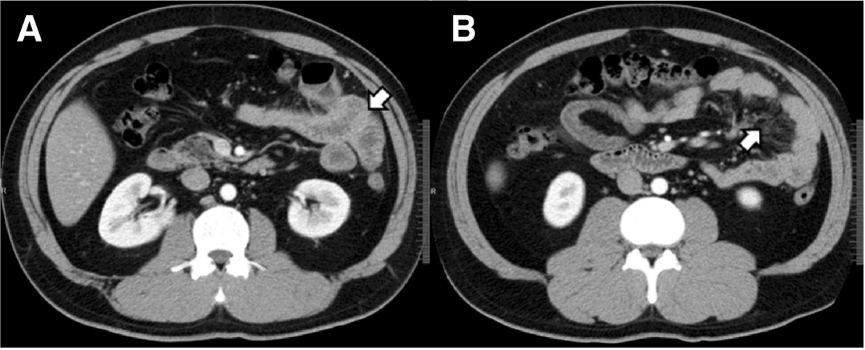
Findings of abdominal computed tomography (CT). A. showing a near-circumferential wall thickening in the jejunum (arrow). B. showing increased density of the mesenteric adipose tissue in the jejunum (arrow).
